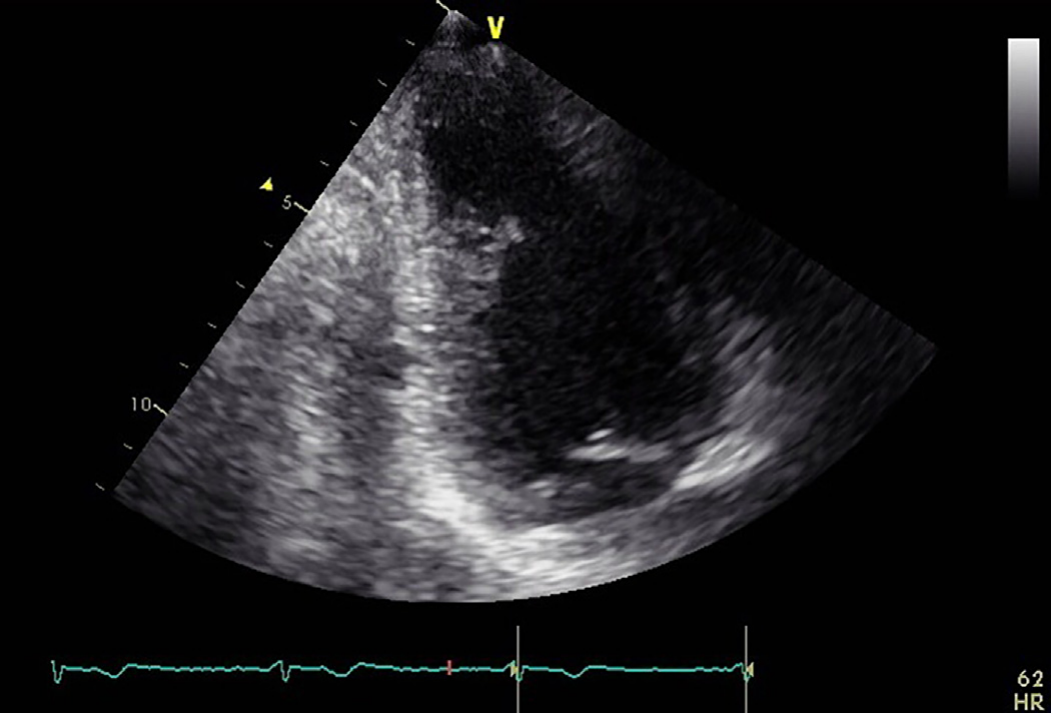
The patient's follow-up transthoracic echocardiography (TTE) showed no remaining thrombi, further supporting the diagnosis after anticoagulant treatment.
